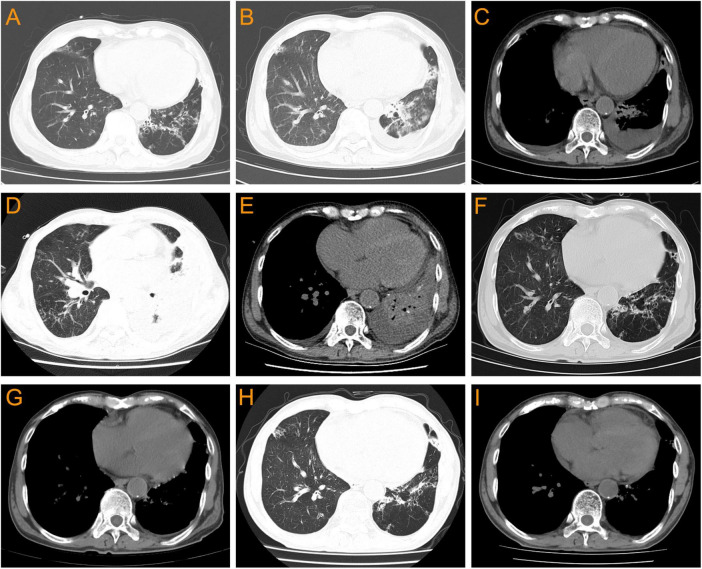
Pulmonary CT changes of this patient before and after *Nocardia* infection. (A) 12 days before admission (with no *Nocardia* infection): bronchiectasis without obvious pulmonary infection or pleural effusion. (B,C) On admission: bilateral bronchiectasis with pulmonary infection and bilateral pleural effusion. (D,E) On the 14th day of admission: worsening of bilateral pulmonary infections and bilateral pleural effusion, accompanied by left atelectasis. (F,G) 12 days after linezolid combined with TMP-SMZ treatment: bilateral pulmonary infection improved significantly, with a small amount of left pleural effusion. (H,I) 2 months after discharge: bronchiectasis with no obvious pulmonary infection and pleural effusion.
